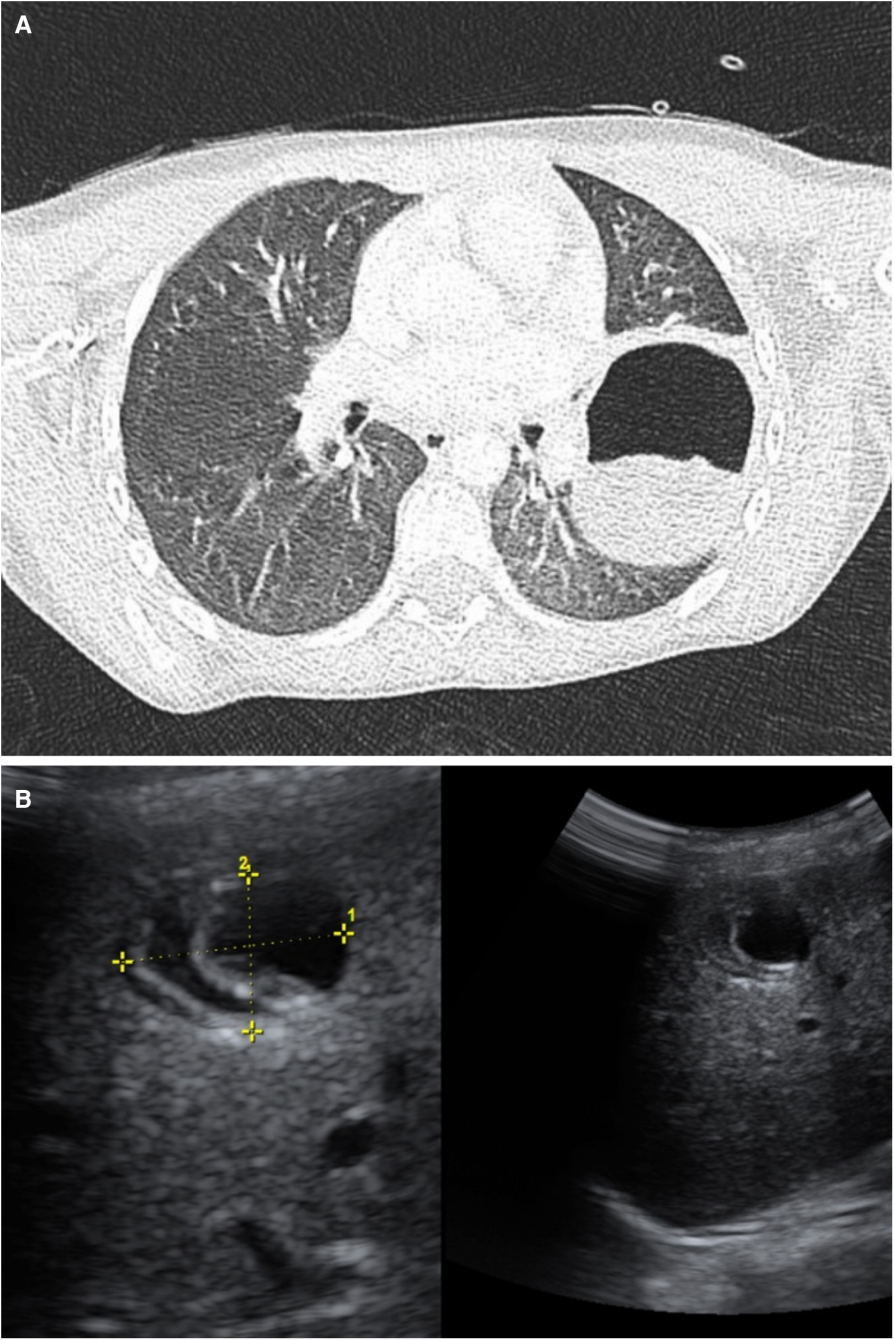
(A) Chest CT. Left lower lobe: A round, heterogeneous, hypodense image is denoted with a hyperdense halo, measuring 52 × 64 × 81 mm. Suggestive of a cyst. Bilateral diffuse ground glass opacity pattern observed in the lungs. (B) Abdominal Ultrasound: The liver is in a normal position with preserved echogenicity. A cyst-like lesion is observed in segment VIII, containing echogenic membranes, measuring 23 × 21 × 20 mm, and appearing avascular. Consistent with hydatid cyst.
